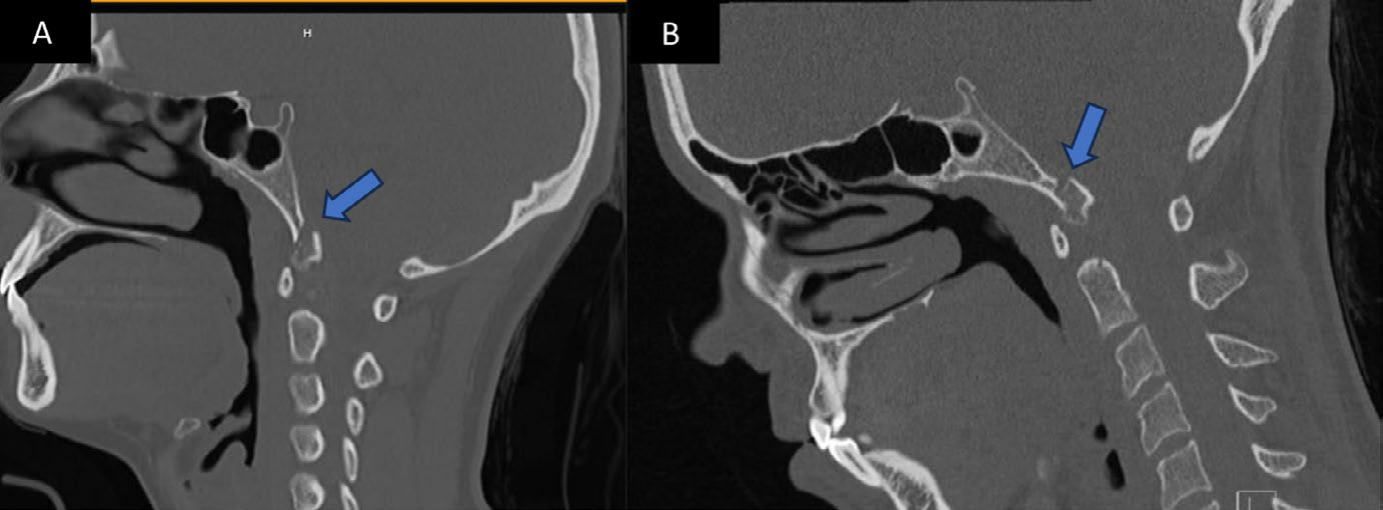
A and B non‐contrast CT maxillofacial (sagittal view) showing initial avulsion type fracture through right occipital condyle, and 3 months follow up CT in a similar plane showing callus formation.
